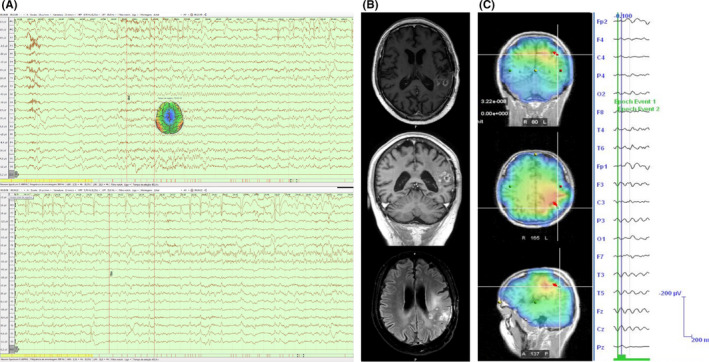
(A) EEG of painful seizure, which starts with diffuse attenuation of electrogenesis, followed by rhythmic theta activity (left fronto‐centro‐parietal and midline) with progressive slowing until the end of the painful episode; (B) Brain MRIs (T1 + gad and flair sequences) showing local tumor recurrence; (C) sLoreta (fast Fourier transform approximation) for the first seconds of the rhythmic theta activity. Inverse solution with a left parietal localization. ASA 4.7 software with standard MRI and electrode digitalization for the 10/20 system
